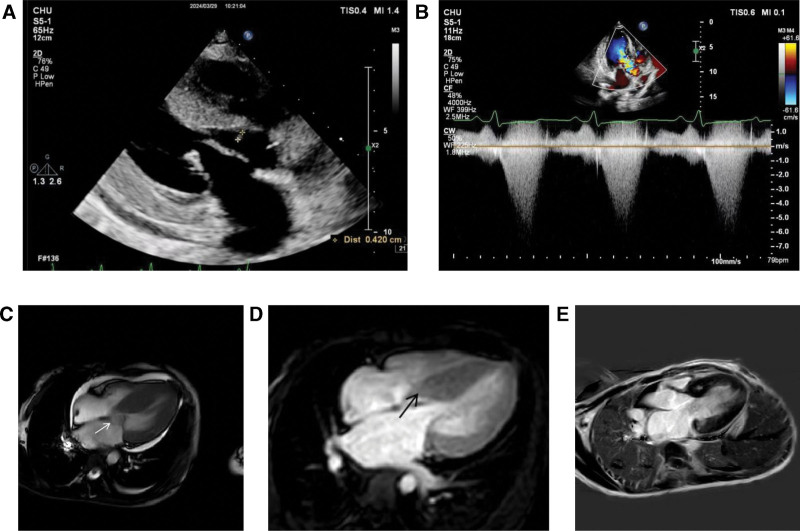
Echocardiogram and magnetic resonance imaging of the patient. (A) An echocardiogram shows vegetation (++) occupying the left ventricular outflow tract. (B) Echocardiogram shows left ventricular outflow tract obstruction. (C) MRI showed significant thickening of the ventricular septum and adjacent inferior wall and systolic mitral valve with SAM sign (white arrow). (D) First overperfusion showed a small lamellar area of reduced perfusion in the left ventricular septal wall (black arrow). (E) Delayed enhancement showed multiple focal intensifications in the interventricular septum. MRI = magnetic resonance imaging, SAM = systolic anterior motion.
